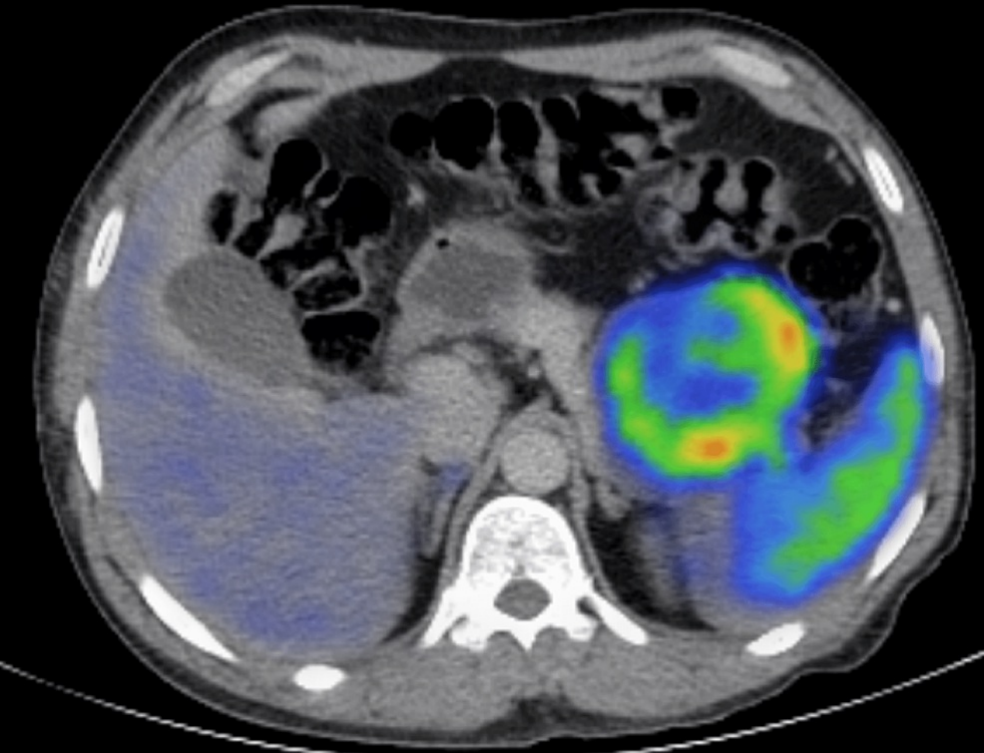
Ga-68 DOTA PET/CT pre-operative image showing pancreatic mass with increased uptake DOTA - dodecane tetraacetic acid, PET - positron emission tomography
